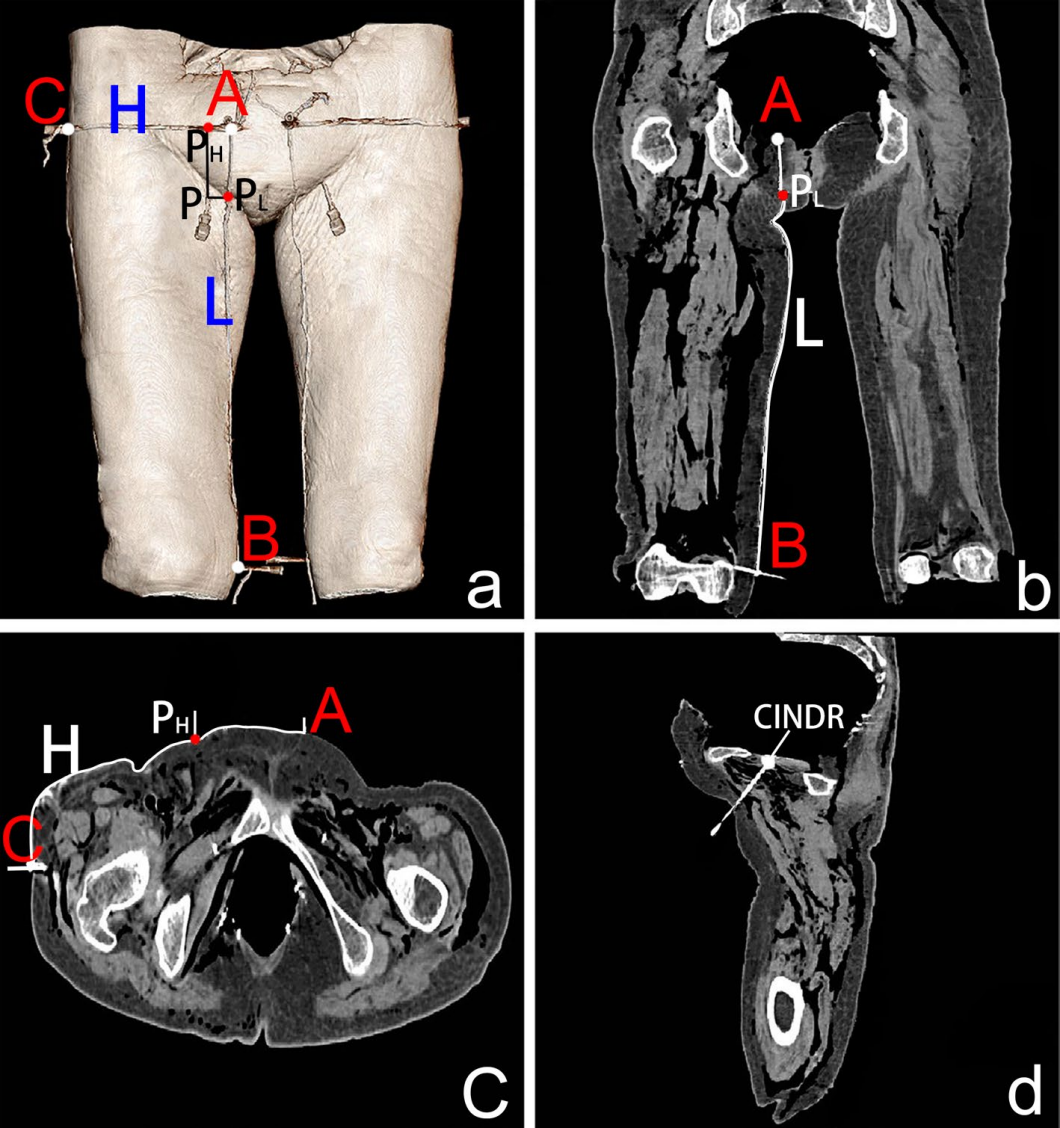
Spiral CT localization image of right obturator internus muscle CINDR (female). a CINDR body surface projection position and the designated reference line. A = pubic tubercle, B = medial epicondyle of femur, C = greater trochanter, P = projection point of CINDR in front of the thigh. P_H_ is the intersection of a vertical line through P with line H, P_L_ = intersection of a horizontal line through P with line L, A-P_H_ = H′, A-P_L_ = L′. b The length of the L and L′ lines was measured through the pubic tubercle and coronal section of the medial epicondyle of the femur. c The length of the H and H′ lines was measured on the cross-section of the pubic tubercle and the greater trochanter connection. d The depth of the CINDR was measured through the sagittal plane of the P point
